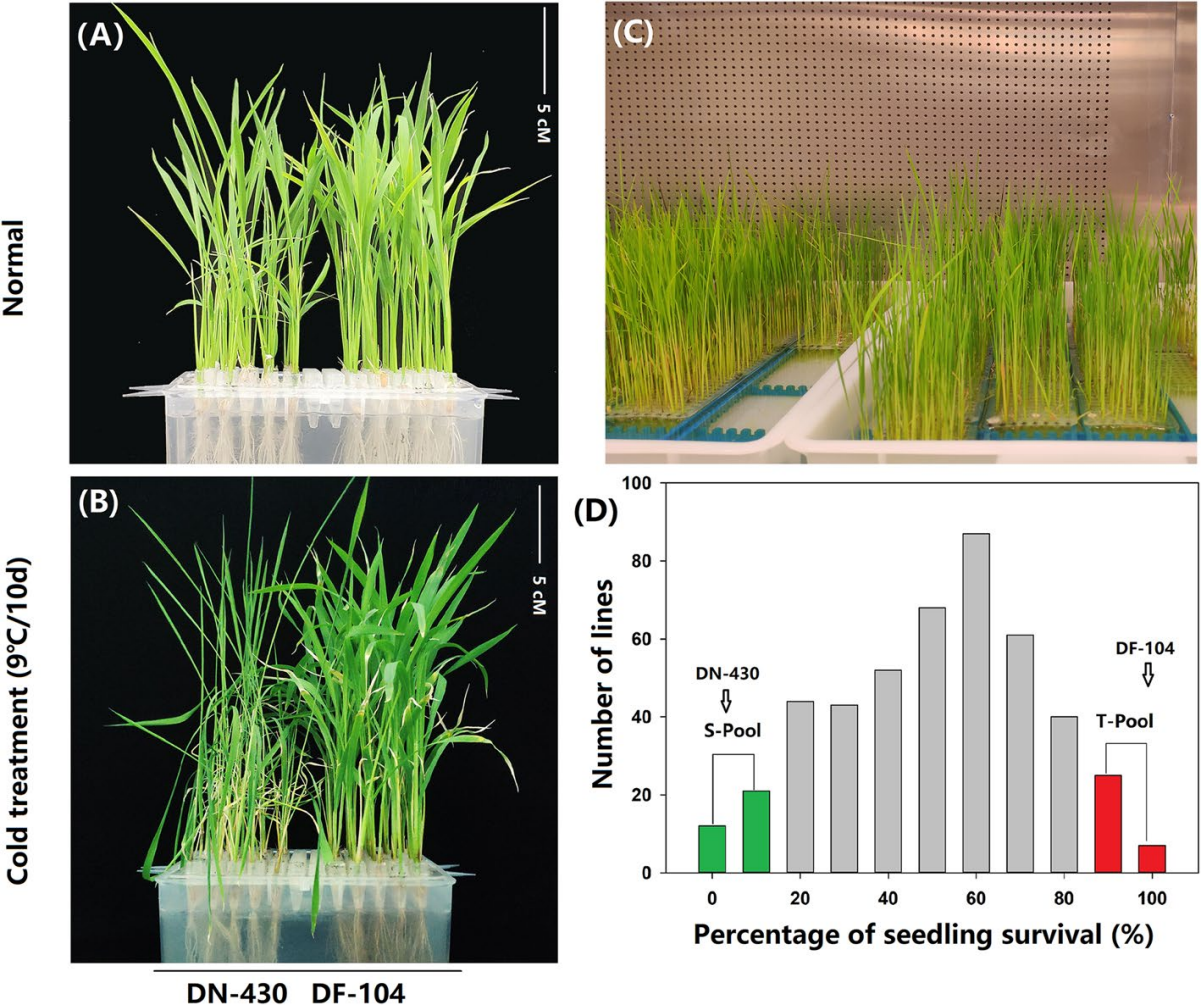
Phenotype analysis of CTS in rice. A Performance of DN430 and DF104 under normal conditions. B Performance of DN420 and DF104 under cold treatment (9°C/10 days). C Identification of CT in the F2 population in an artificial climate chamber. D Frequency distribution of SR variation measured among 460 mapping individuals of F2:3 populations
